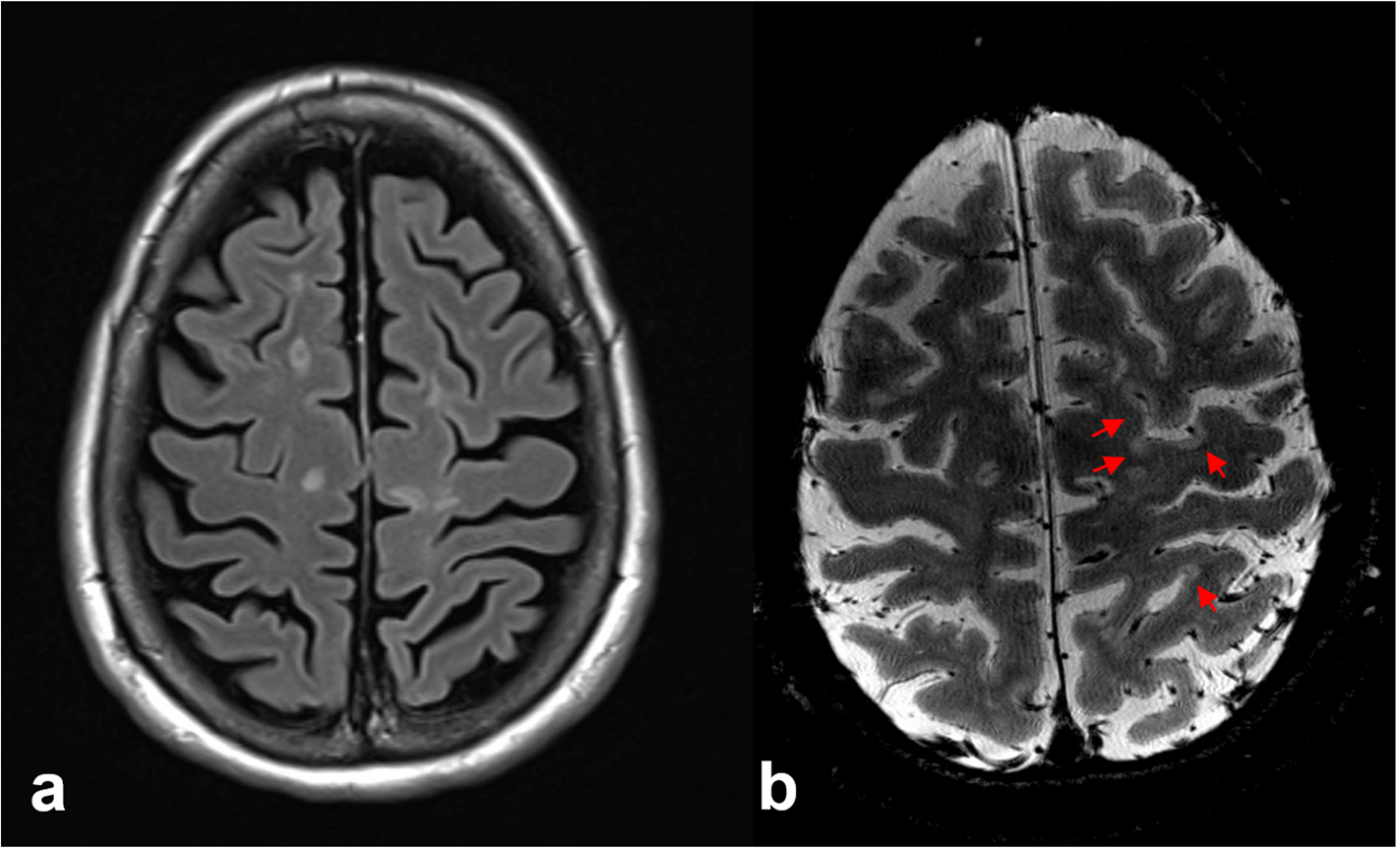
Cortical lesions in multiple sclerosis on 3-T and 7-T magnetic resonance imaging. Axial fluid-attenuated inversion-recovery (FLAIR) image acquired at 3 T (a) and axial T2*-weighted image acquired at 7 T (b). The 7-T image shows many more cortical lesions (red arrows), which are not visible on the axial FLAIR (a) or T2-weighted image (not shown) at 3 T, due to a combination of increased contrast and spatial resolution at 7 T
